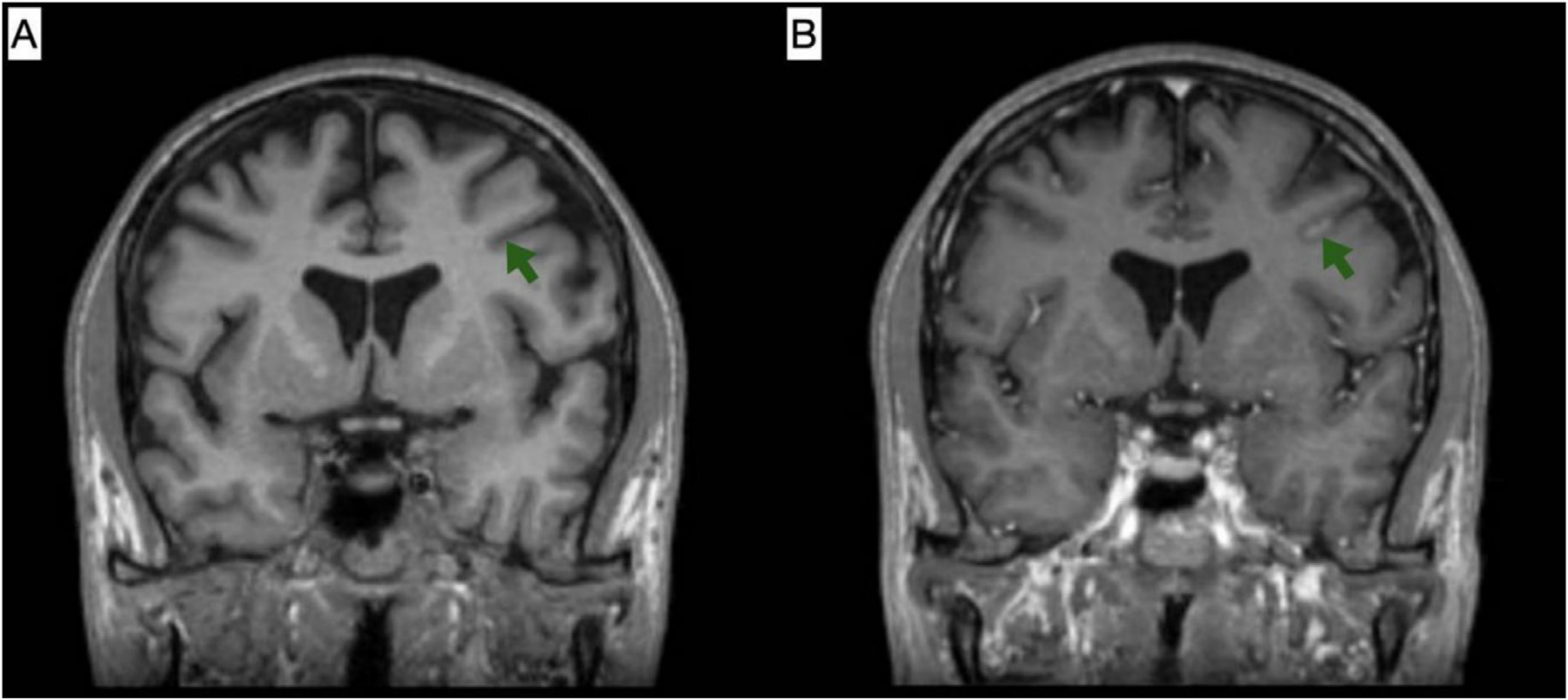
(A) T1-weighted image; (B) T1-weighted image post-contrast. Selected coronal MRI images reveal supratentorial leptomeningeal enhancement of the left cerebrum (indicated by green arrows), suggestive of metastatic deposits.
